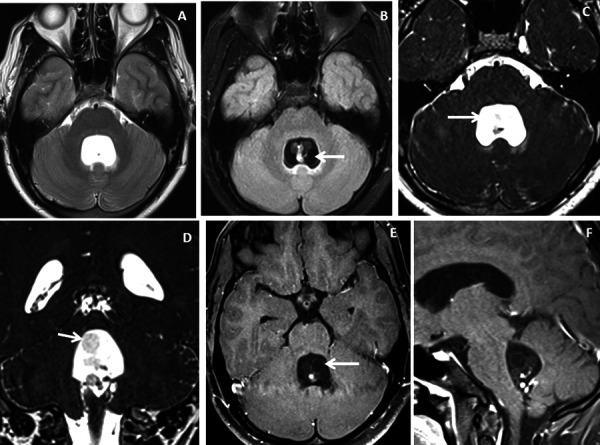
Brain magnetic resonance imaging of 25 years male with headache. (A-B) Axial T2WI and FLAIR images show distension and dilatation of the fourth ventricle with minimal periventricular CSF seepage. Variable signal intensity cystic lesions are seen within the fourth ventricle on the FLAIR image (arrow). (C-D) Axial and coronal heavily T2W images show multiple thin-walled hyperintense cystic lesions (arrows) with less hyperintense CSF signal within the 4^th^ ventricle with variable sizes irregular nodules within. (E-F) Axial and sagittal T1W post-contrast image shows thin smooth enhancement of the intraventricular cyst (arrow) with enhancing irregular nodules. Post-contrast enhancement is also seen along the ependymal lining of the 4^th^ ventricle, in posterior and inferior aspects.
